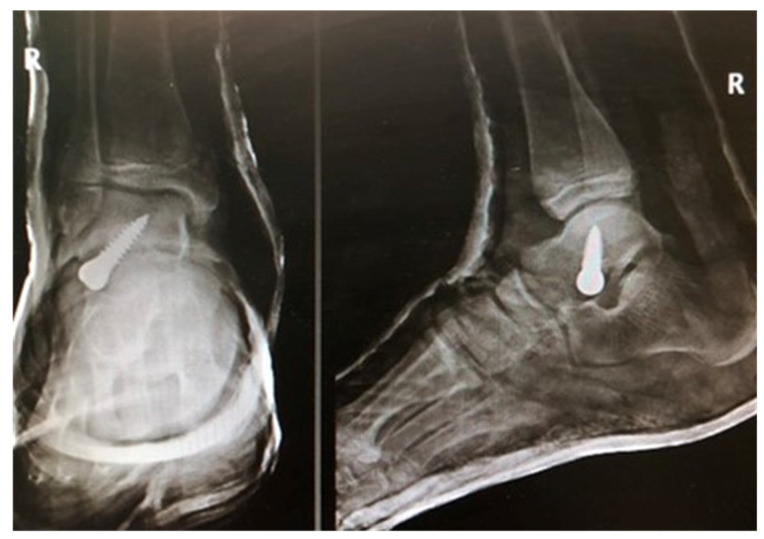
Radiographic images after pes planovalgus correction with a Spherus screw.
